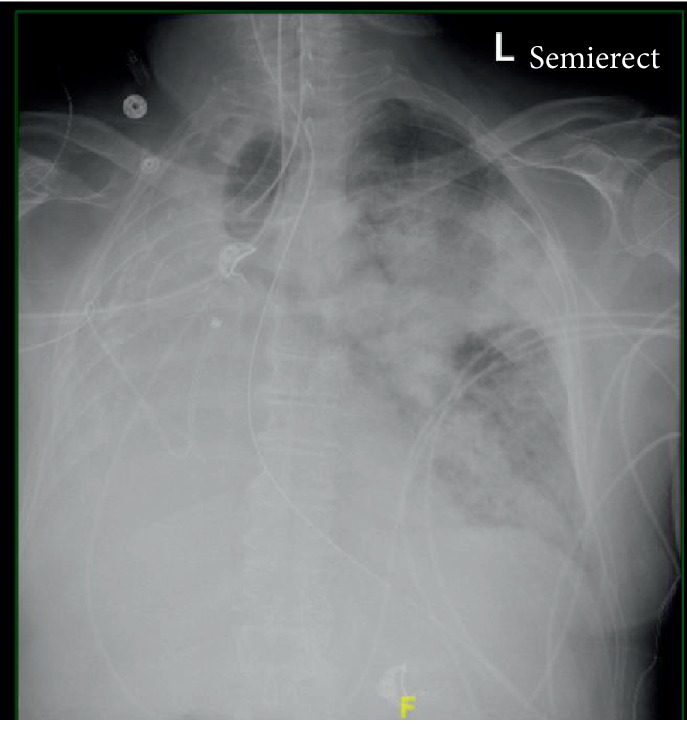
Chest X-ray done after advancement of the endotracheal tube, shown in the image to be projected toward the right side of the trachea. Persistent airspace disease noted in the left upper lobe.
